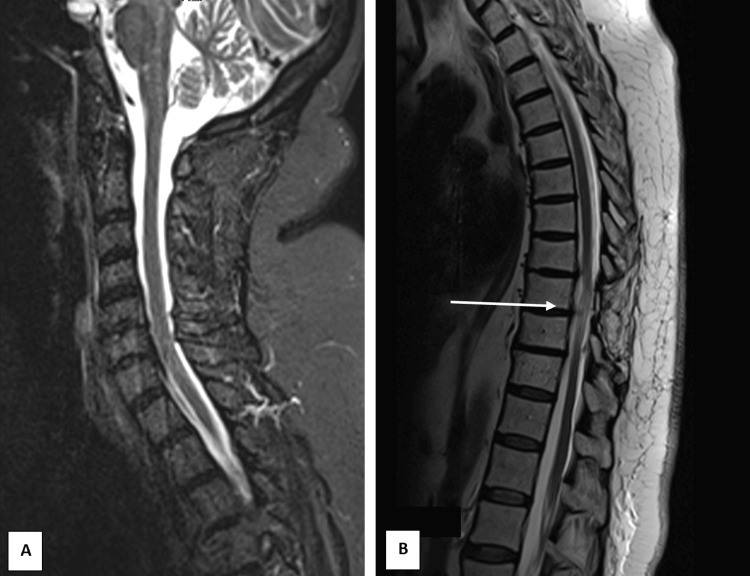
Sagittal magnetic resonance imaging scans six months after treatment. The images (A) cervicodorsal region and (B) thoracolumbar region demonstrate residual signal enhancement at the site of the bilateral laminectomy defect at the T9 level (arrow), consistent with chronic changes. No additional abnormalities are noted.
